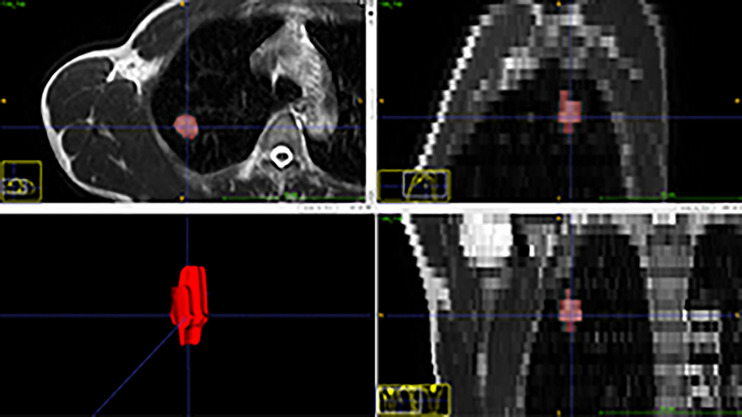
Segmentation of a nodule in the upper right lobe on T2-weighted images.
